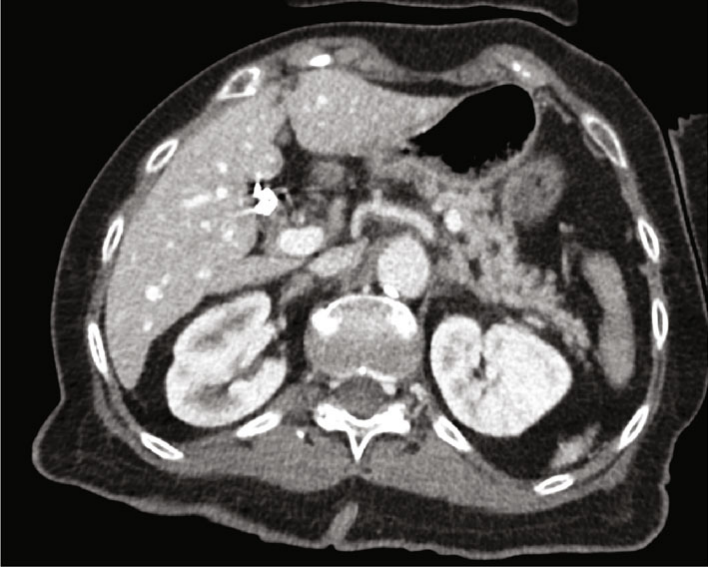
Axial computed tomography image of the upper abdomen obtained on admission demonstrates normal attenuation and enhancement of the liver parenchyma, hepatic veins, and portal vein branches with no evidence of portal venous gas identified. The remainder of the CT abdomen and pelvis exam was negative for source of infection to explain the patient's sepsis clinical presentation.
